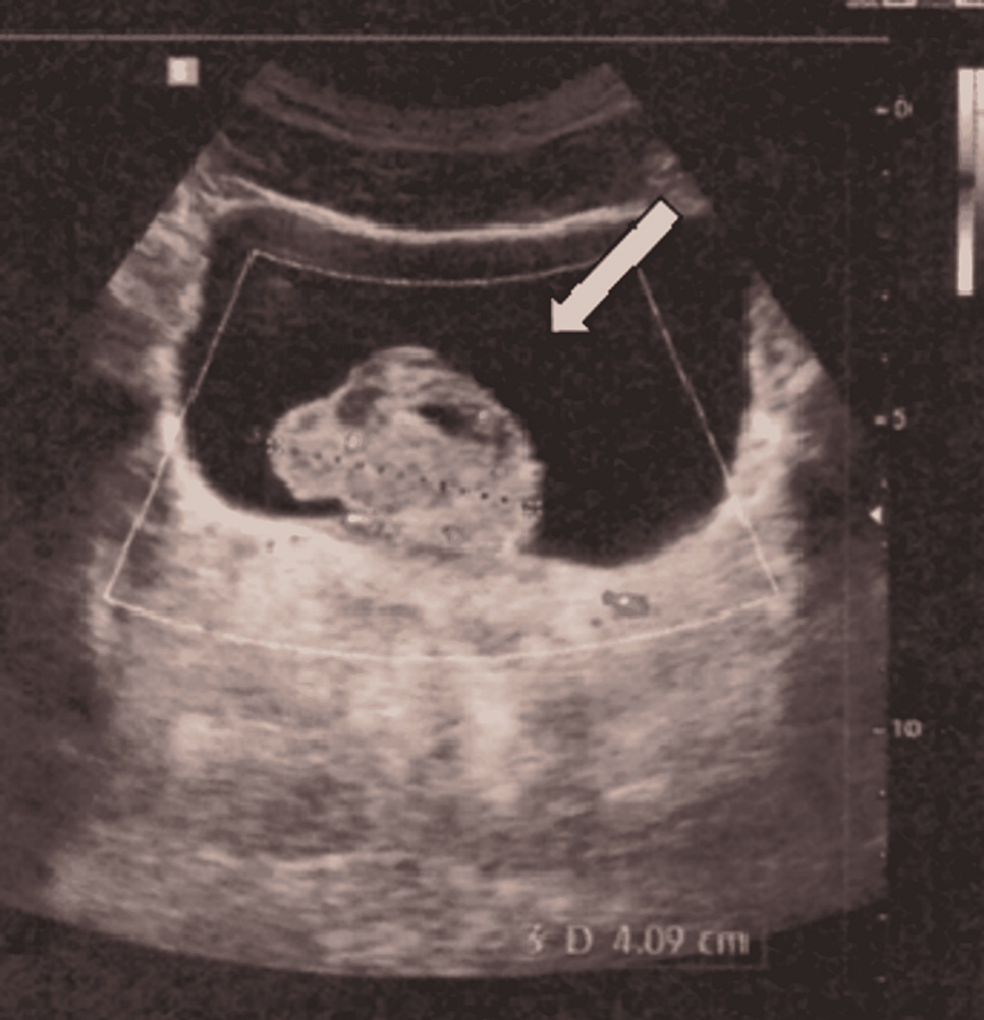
Ultrasonography showing a bladder lesion (white arrow) in the posterior wall with signs of neoplasia.
